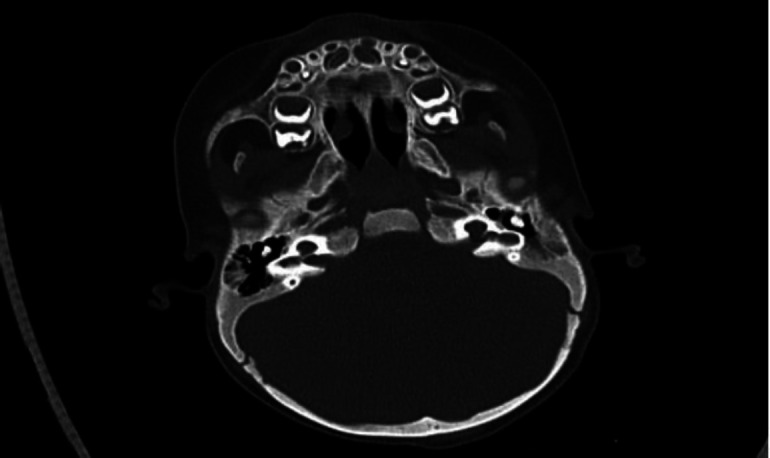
High resolution computed tomography temporal bones showing malformation of inner ear bilaterally (marked by black arrows)
